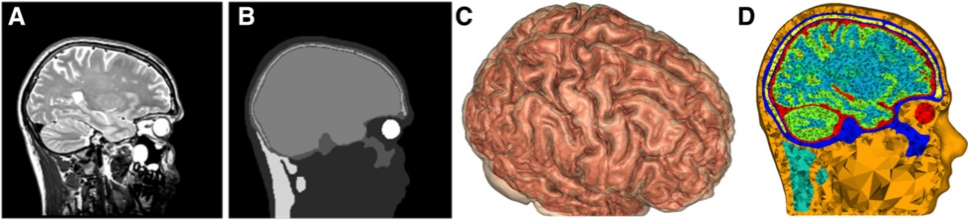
Realistic head model: (A) A sagittal cut plane of the T2 weighted MRI showing the different skull layers. (B) The same sagittal cut plane of the manually corrected segmentation including skin, skull compacta, skull spongiosa, neck muscle, eyes and one compartment for inner skull (CSF, GM and WM, before segmentation with Freesurfer). (C) High resolution triangular surface meshes of GM (transparent) and WM (red), constructed with Freesurfer. (D) Sagittal cut plane of the final tetrahedral volume mesh created with TetGen. The different tissue types are represented with different colors.
